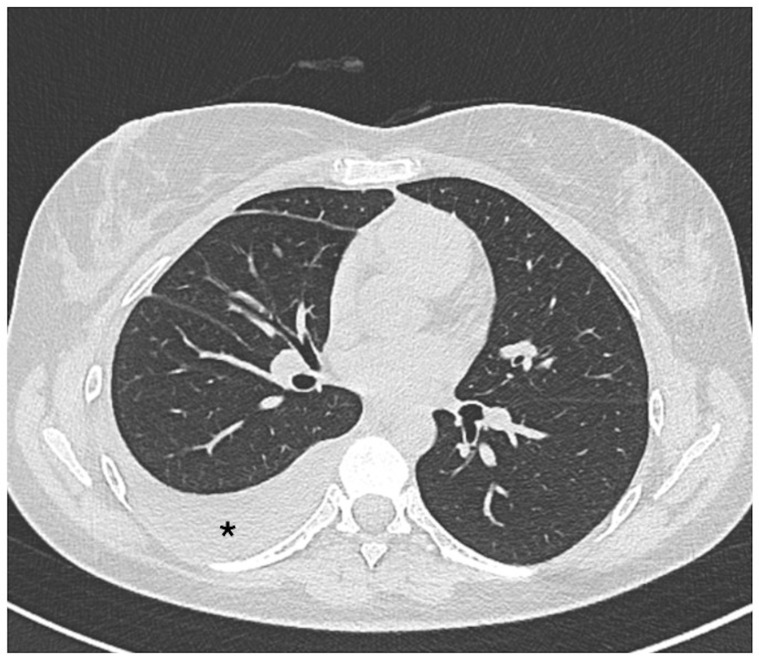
Axial non-contrast thorax computed tomography scan in a patient with systemic lupus erythematosus. The exam revealed abnormal accumulations of fluid within the pleural space (asterisk).
